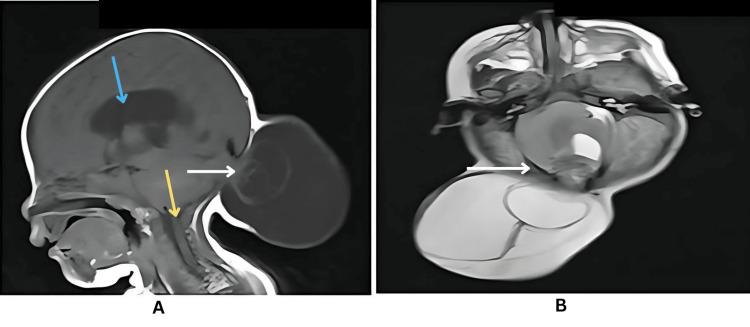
A. MRI sagittal T1-weighted sequence of the brain in Type 3 Chiari malformation showing herniation of posterior fossa contents, including the cerebellar hemisphere and meninges, through a defect (white arrow) in the occipital calvaria. There is downward displacement of the cerebellar tonsil through the foramen magnum (yellow arrow), along with supratentorial ventriculomegaly (blue arrow). These features are suggestive of encephalocele. B. MRI T2-weighted axial sequence of the brain in Type 3 Chiari malformation showing herniation of posterior fossa contents, predominantly the left cerebellar hemisphere and meninges, through a defect in the right occipital calvaria (white arrow). The posterior fossa appears crowded with downward displacement of the right cerebellar tonsil through the foramen magnum, along with supratentorial ventriculomegaly.
